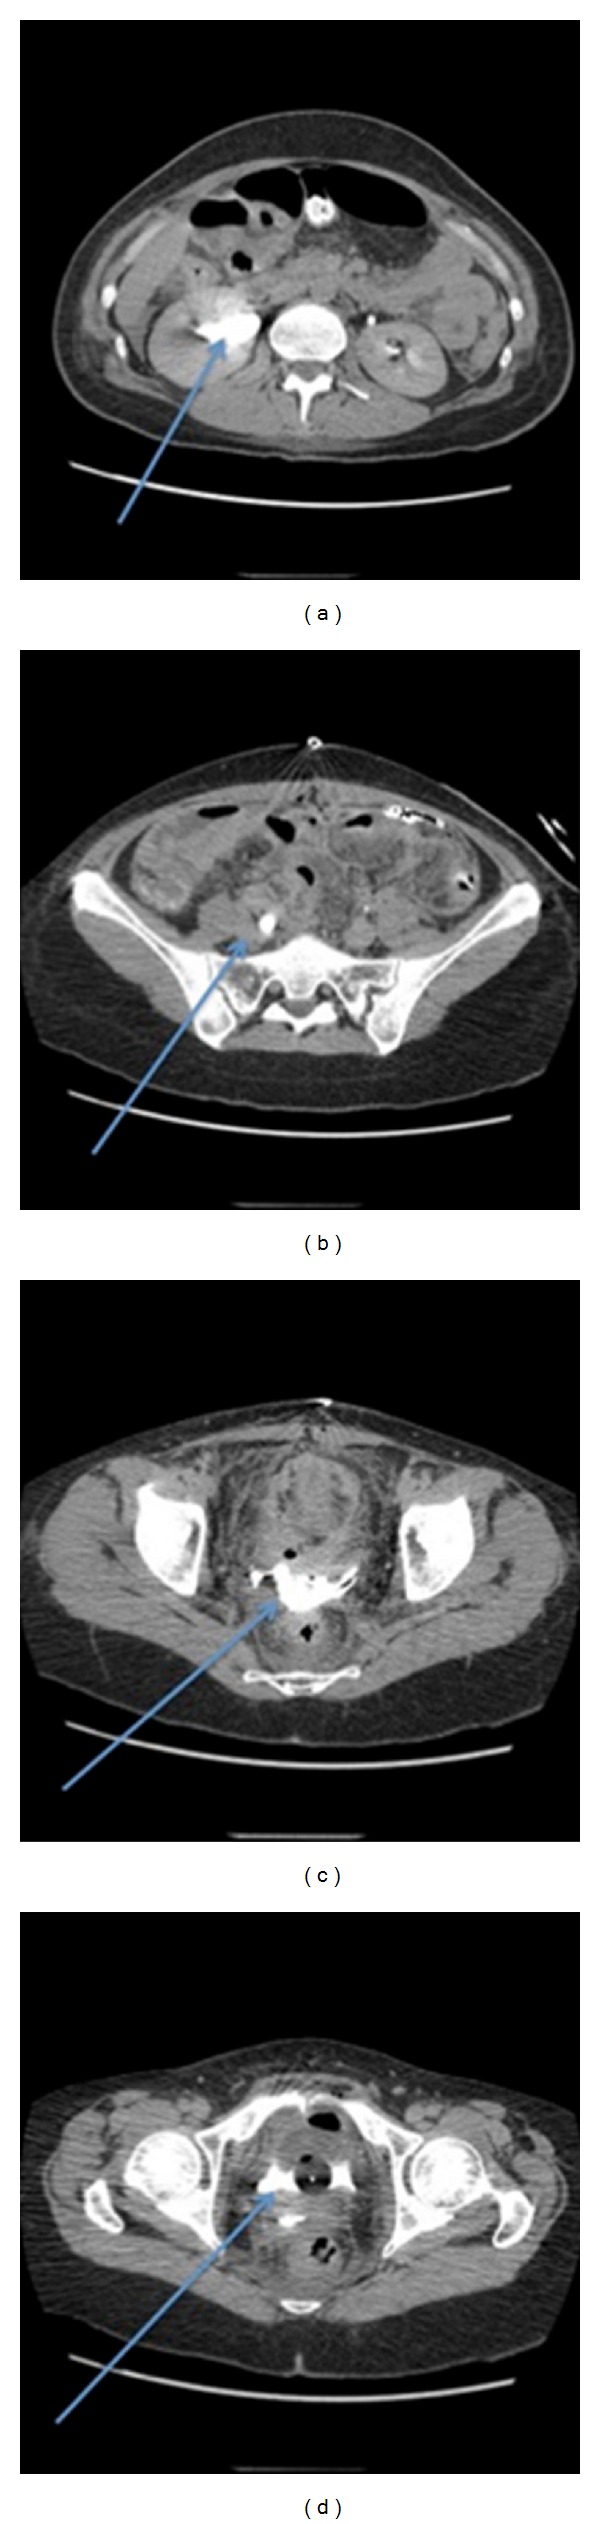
CT scan of the abdomen and pelvis on postoperative day 17. (a) Excretory phase. The arrow shows dilatation of the right pyelocaliceal cavities. (b) Dilatation of the right ureter. (c) and (d) Urinoma with extravasation of contrast agent into the pelvis.
